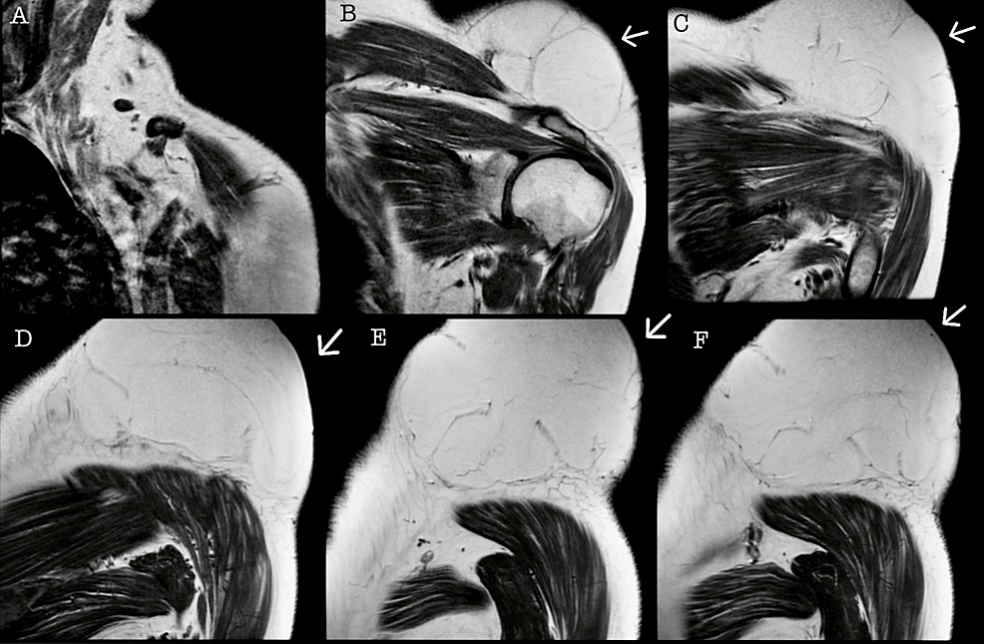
Magnetic resonance imaging in coronal section in T1 The arrow shows a hyperintense, well-defined, extrafascial tumor, without signs of bone erosion or muscular infiltration and joint involvement.
